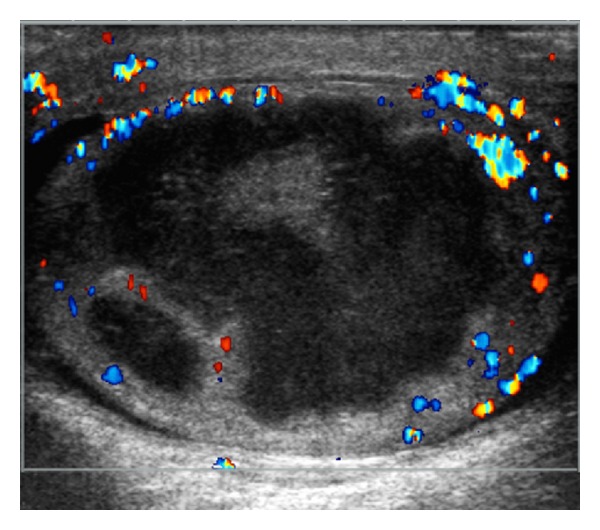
Scrotal ultrasound at presentation.
